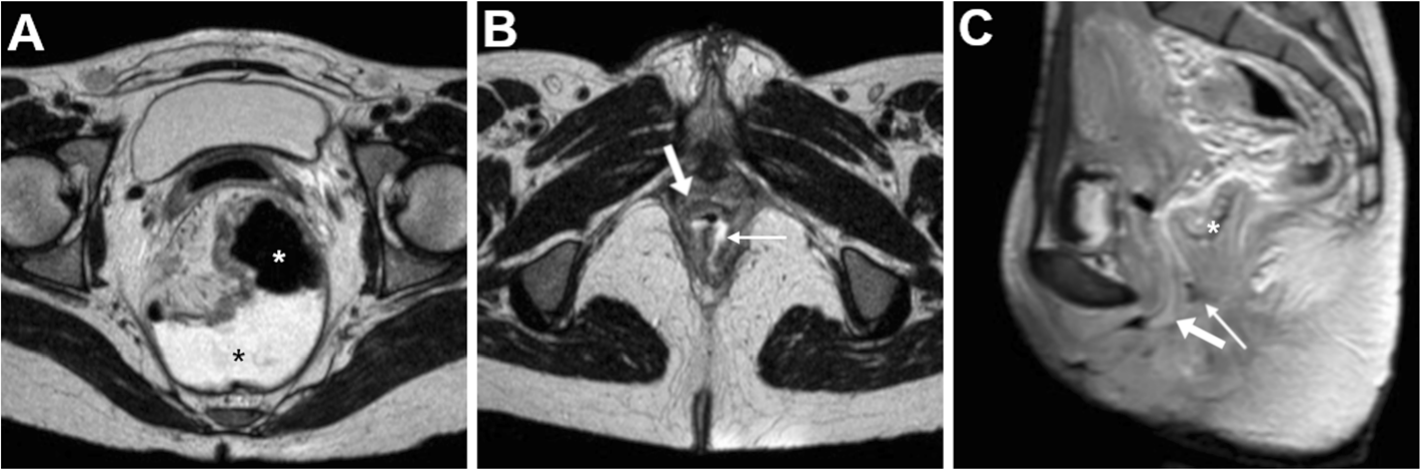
Ano-VF following total proctocolectomy with ileal pouch-anal anastomosis (IPAA) in a 44-year-old with ulcerative colitis. Axial T2-weighted (a, b) and sagittal post-gadolinium T1-weighted (c) MRI images show fluid distension of the ileal pouch (*) and a small midline fistula (thin arrows) connecting the IPAA to the vagina (arrow)
